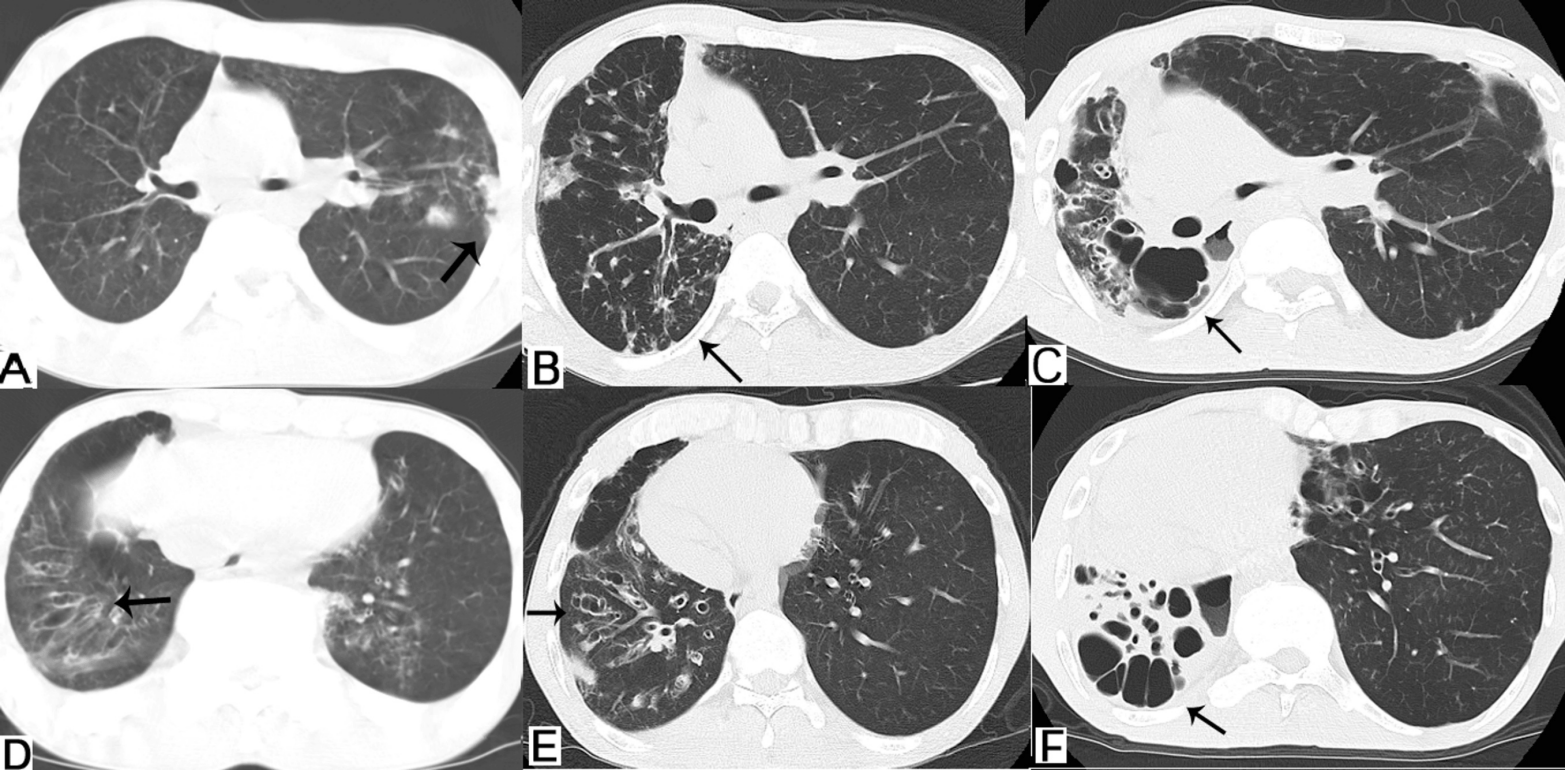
retrospective findings on serial chest CT taken at the age of (A, D) 15 years, (B, E) 22 years, and (C, F) 29 years (A) Mild infiltration (black arrow) in the upper regions on the left side of the lung; (B) Mild bronchiectasis and infiltration (black arrow) in the upper regions on the right side of the lung; (C) Severe bronchiectasis and cyst (black arrow) in the upper regions on the right side of the lung; (D) Cylindrical bronchiectasis (black arrow) in the lower regions on the right sides of the lung; (E) Beaded bronchiectasis (black arrow) in the lower regions on the right side of the lung; (F) Consolidation and multiple cysts (black arrow) in the lower regions on the right side of the lung.
